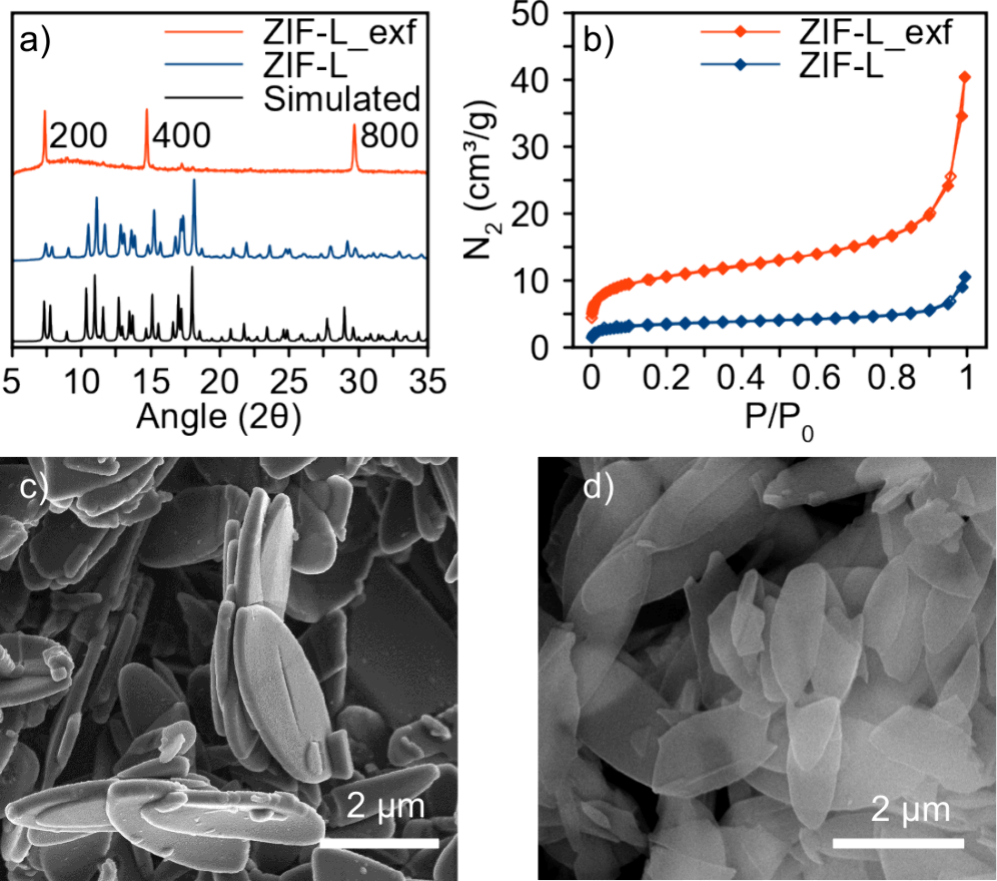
Characterization of ZIF-L and ZIF-L_exf: (a) Powder X-ray
